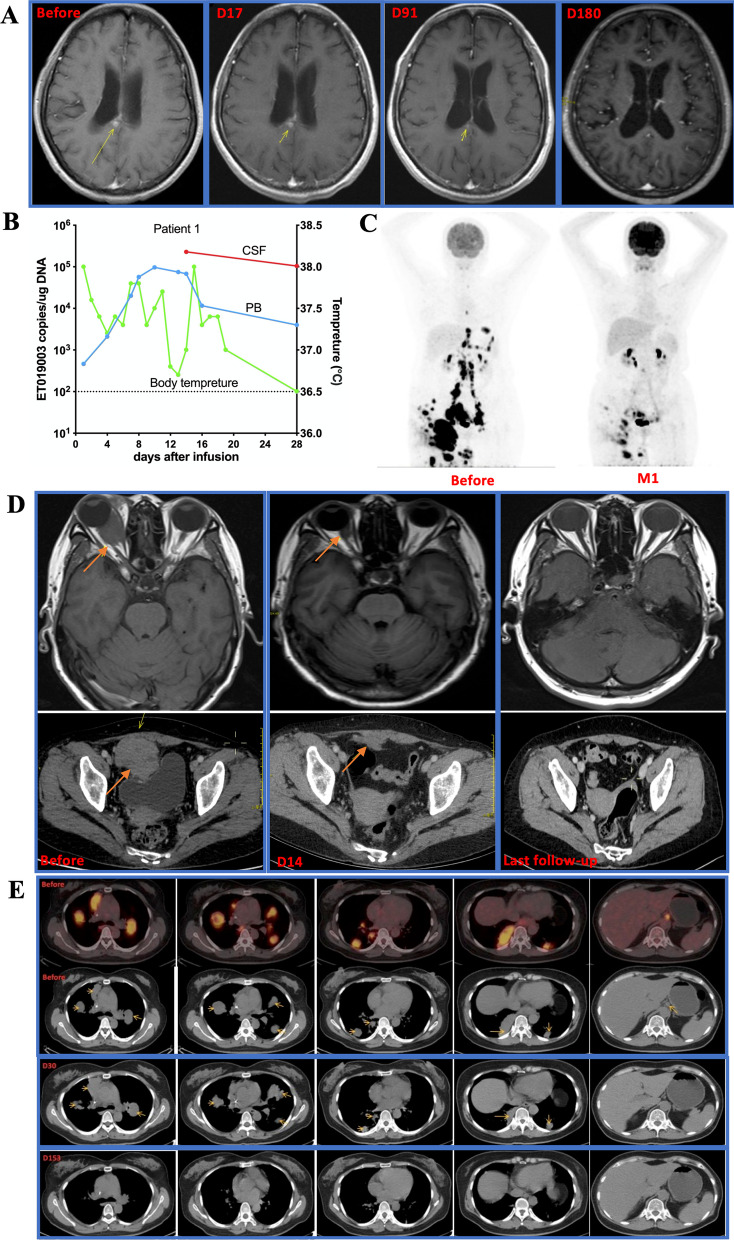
Clinical responses of ET019003 cells. A Changes in cranial magnetic resonance imaging (MRI) scans of patient 1. B ET019003 copies per microgram (µg) of genomic DNA in peripheral blood (PB) and cerebrospinal fluid (CSF) and body temperature changes in patient 1 within 1 month after infusion. C Changes in positron emission tomography-computed tomography (PET-CT) scans of patient 2. D Changes in ocular enhanced MRI and abdominal-enhanced CT of patient 3. E Changes in PET-CT scans of patient 7
